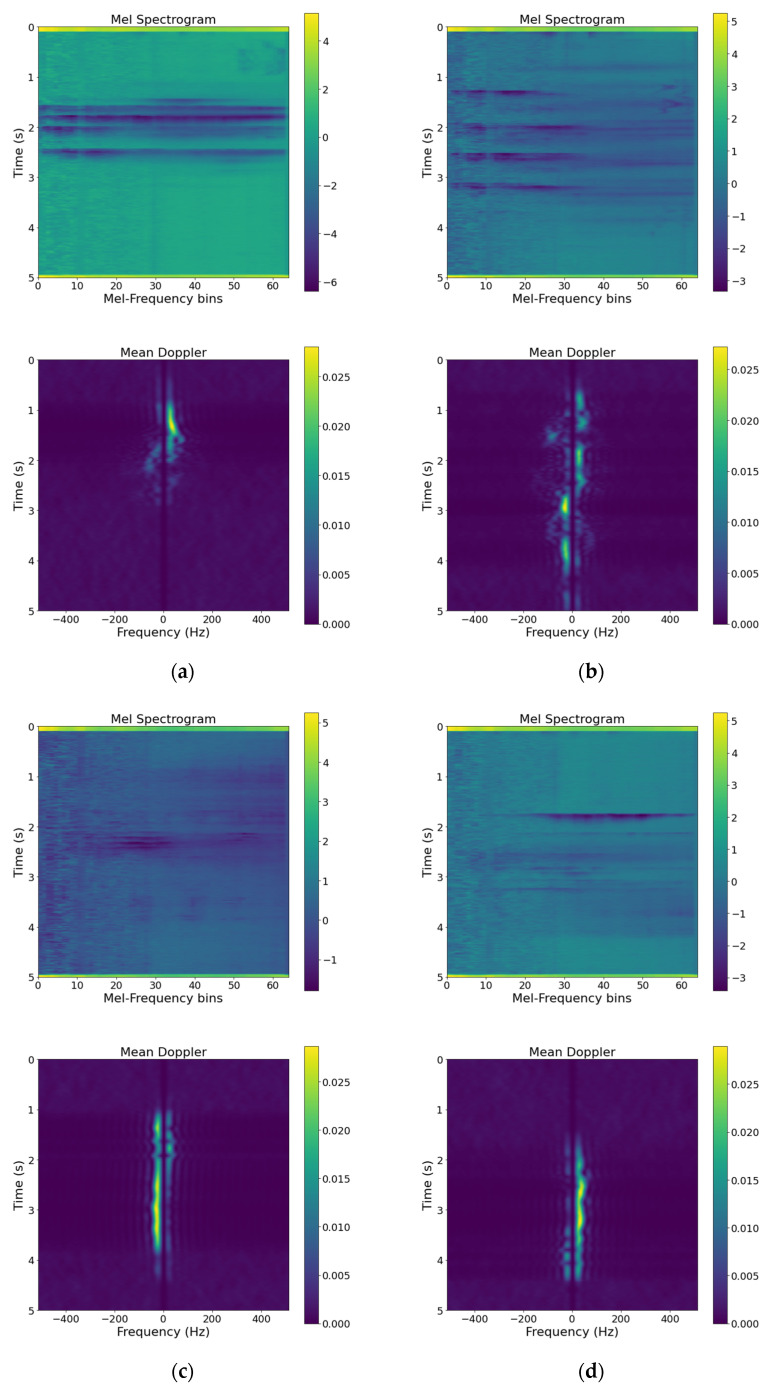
Extracted Doppler and Mel-spectrogram features from recorded data of 4 activities: (a) falling activity; (b) walking activity; (c) sitting activity; (d) standing up activity.
